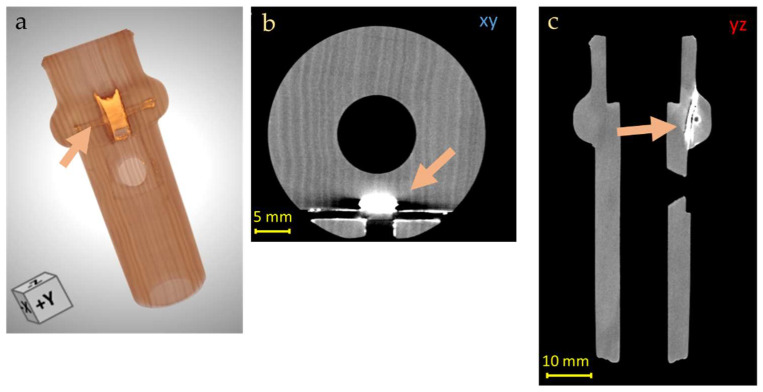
3D render (a) and CT horizontal (b) and lateral (c) slices of the Transverse Flute foot, in which the metallic residues from the brass key insertion are observed (orange arrows). In the 3D rendering (a), the metallic residues are also clearly visible in the upper part of the model.
